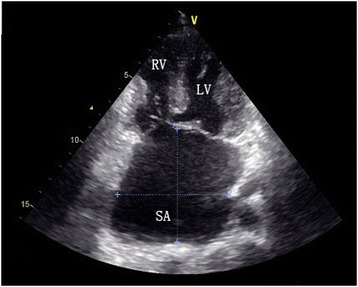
Echocardiography. Echocardiography showed complete absence of interatrial septum. *LV* left ventricle, *RV* right ventricle, *SA* single atrium
